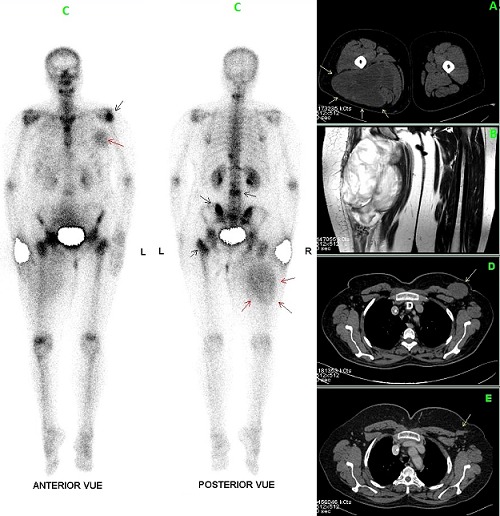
A) intermuscular mass with low-attenuation areas (white arrows) of the right proximal thigh on the CT scan; B) intermuscular mass with marked high signal intensity on T2-weighted MR images, and marked high signal intensity on T2-weighted MR images; C) bone scintigraphy showing multiple hot spots over the proximal epiphysis of the left humerus, sternum, T10-12 and L2-3 and S1 vertebrae, suggestive of skeletal metastases (black arrows) in addition to a soft tissue uptake projected over the right proximal thigh and the left mammary gland corresponding respectively to the primitive tumour (red arrows) and a breast metastasis (red arrow); D) left breast metastasis revealed as a low attenuation area (white arrow) on chest computerised tomography; E) regression of the breast metastasis (white arrow) on a follow-up CT tree years later
